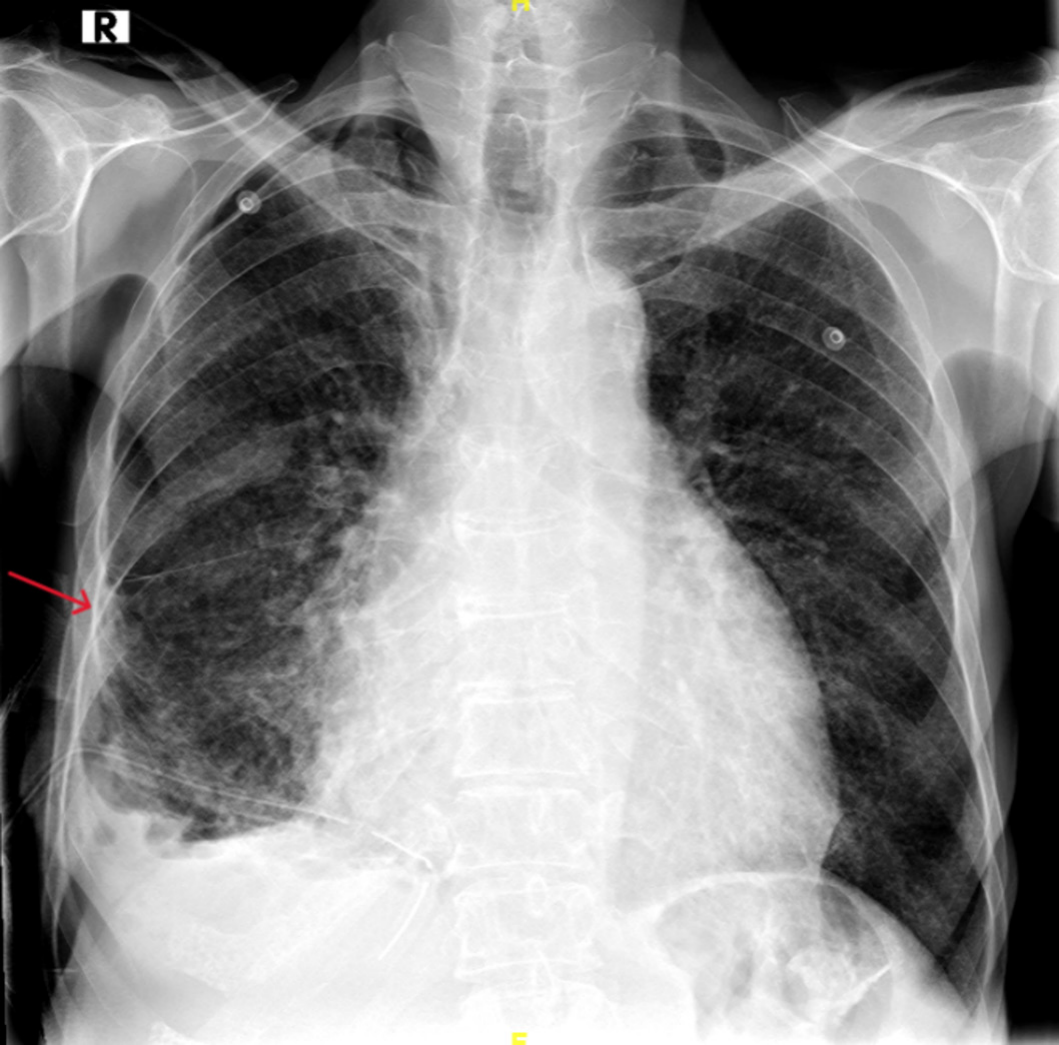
Plain chest radiograph (X‐Ray) post chest tube insertion showing right mid and lower zone consolidation and a blunted costophrenic angle.
